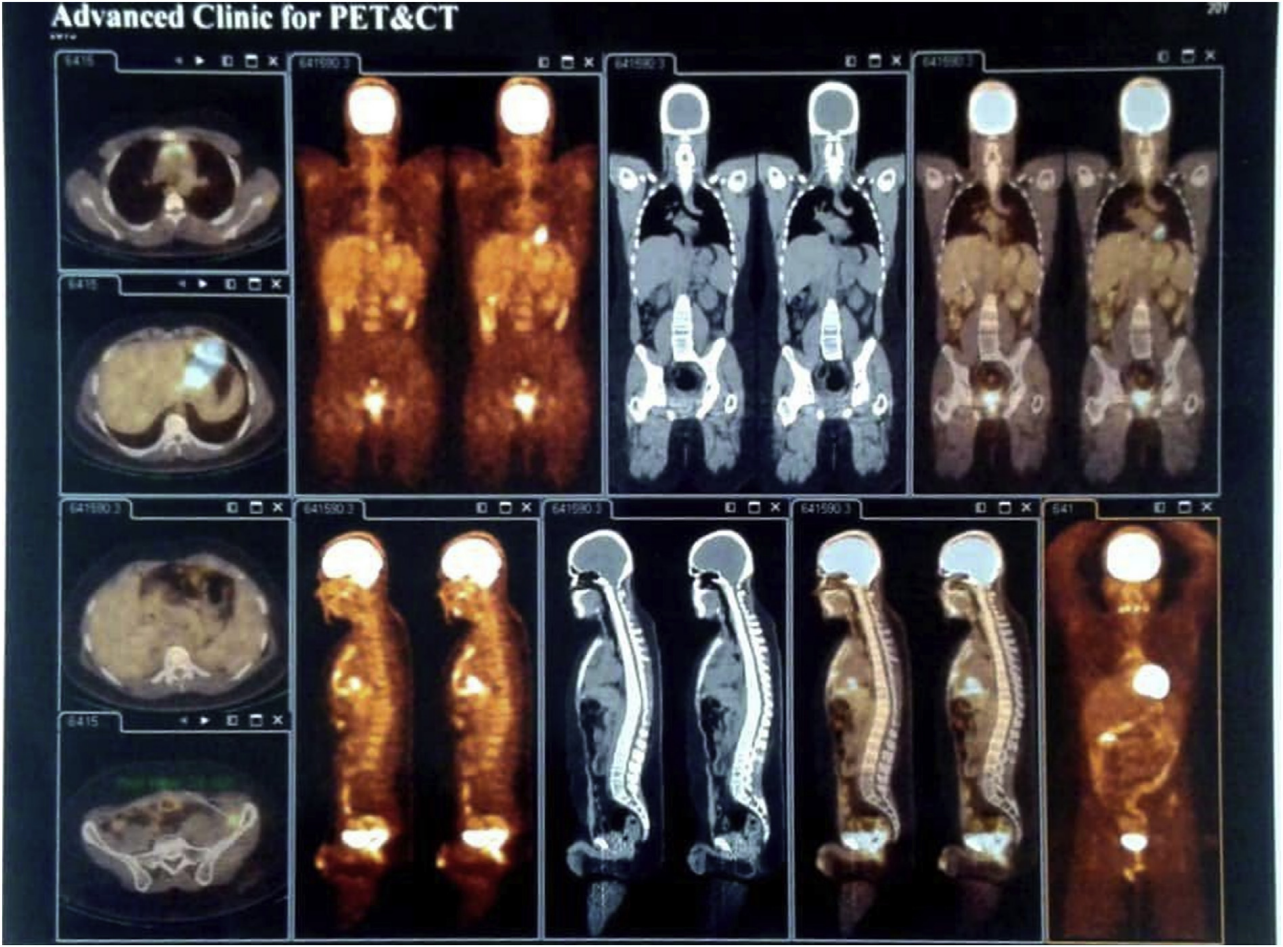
A multislice CT & PET scan after treatment showing small active remnants around the iliac without any other any involvement.
